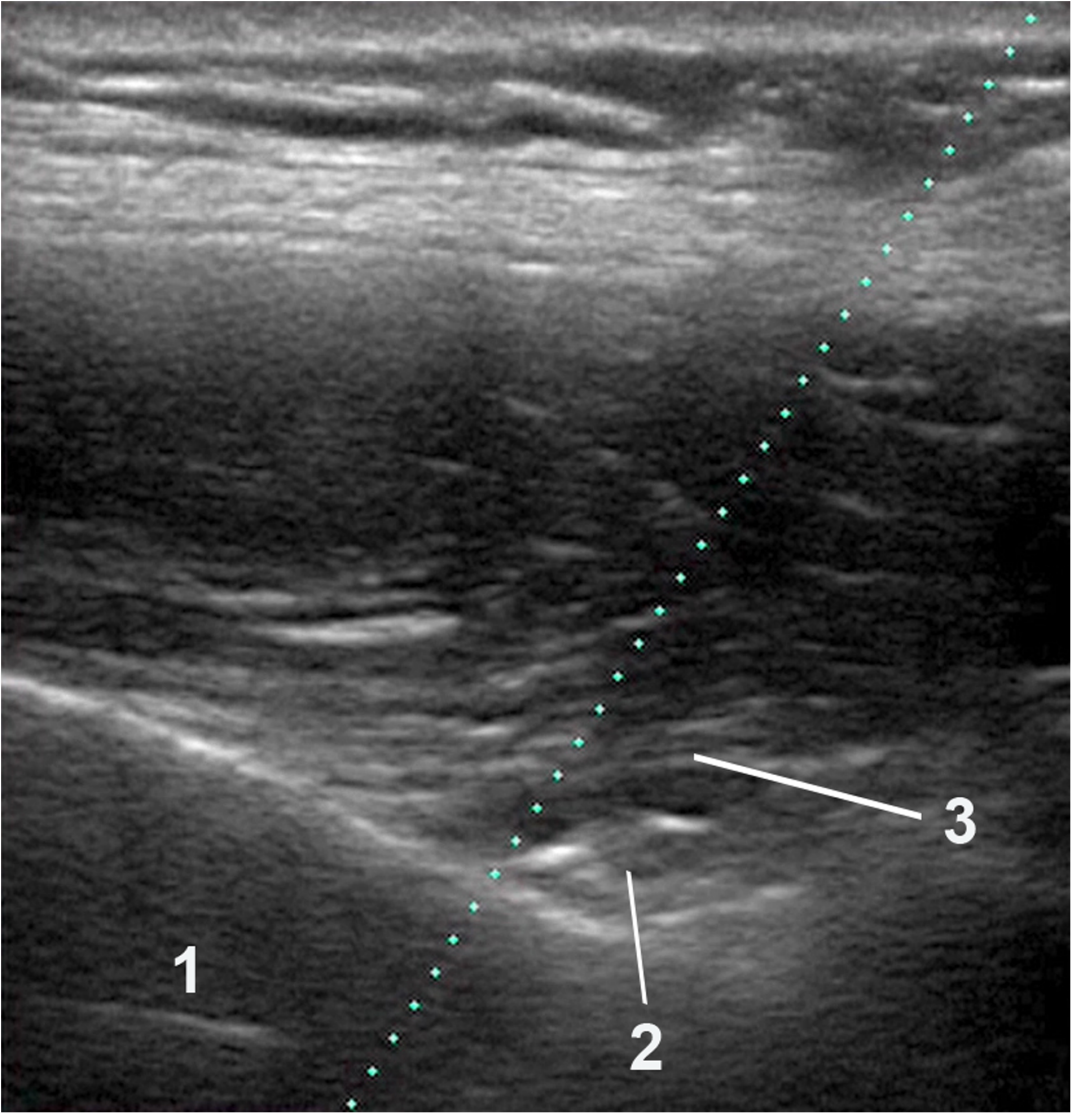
Ultrasound imaging anatomy. Caption: (1) Scapula. (2) Suprascapular nerve. (3) Transverse ligament of the scapula.
